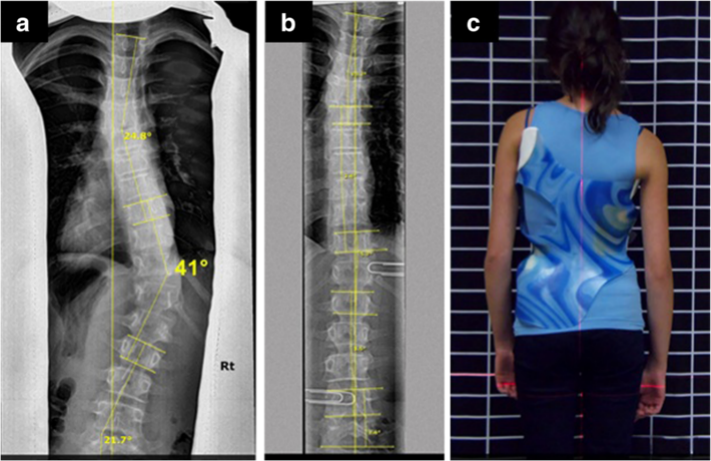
WCR in-brace correction from 41 degrees to 5 degrees Cobb angle. a: The X-ray presents a right thoracic curve of 41 degrees Cobb angle which was measured from T9 to L2 with the curve apex at T11-12. The upper thoracic curve was approximately 25 degrees Cobb angle. b: An in-brace X-ray of patient with Cheneau-Rigo handmade type brace, 18 hours after initial fitting. The thoracic trimline was as high as T10 (above the curve apex) and the pressure pad pushed from T10 to L3, which was above and below the measured Cobb angle. However, the center of maximum pressure was at T-12, as marked by the paperclip in the X-ray. A support force or slight counter force was placed on the left, at the level of L-4 and a left axilla force was applied at the maximum inclined vertebra at approximately T6. This was on the high side, however it provides room for the patient to grow and still provide optimal correction. Care must be taken in these cases to not produce a structural upper thoracic curve. The right thoracic curve reduced in-brace from 41 degrees to 5 degrees Cobb angle and the upper thoracic curve reduced in-brace from approximately 25 degrees Cobb angle to 10 degrees Cobb angle. c: Patient in a Cheneau-Rigo handmade type brace with optimal axilla, thoracic and lumbar pad heights.
